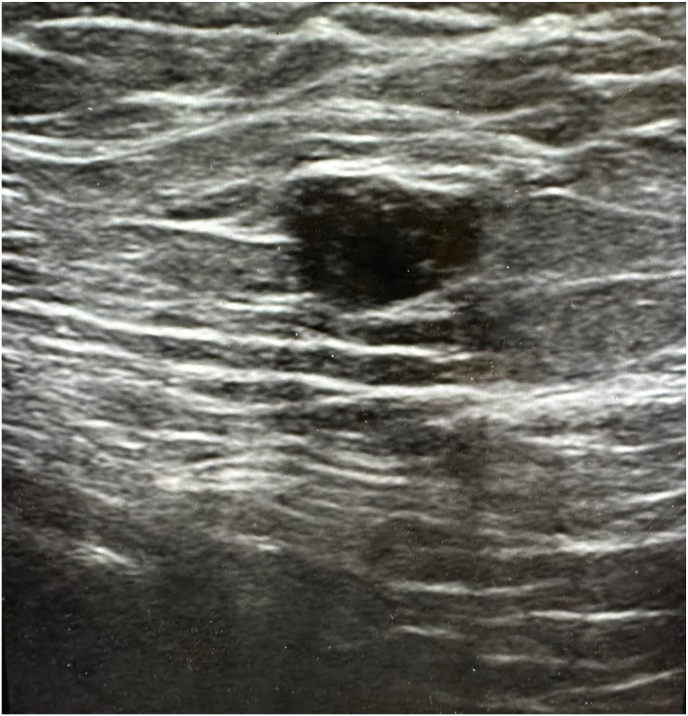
Ultrasound of the right breast.
